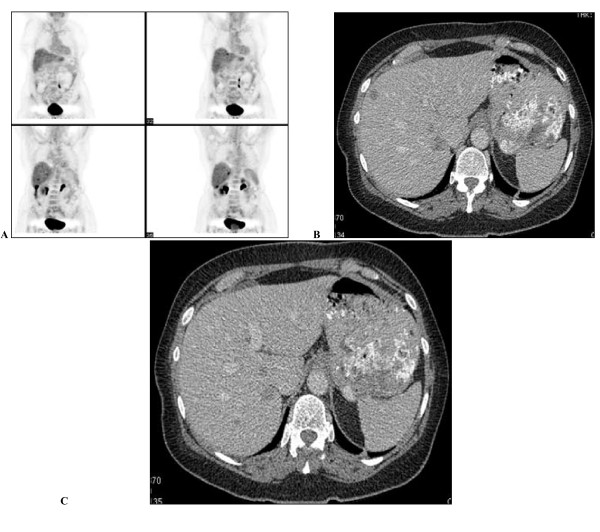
a, b, c – Imaging before primary treatment: multiple liver metastases and no extra hepatic disease described by CT and PET.
